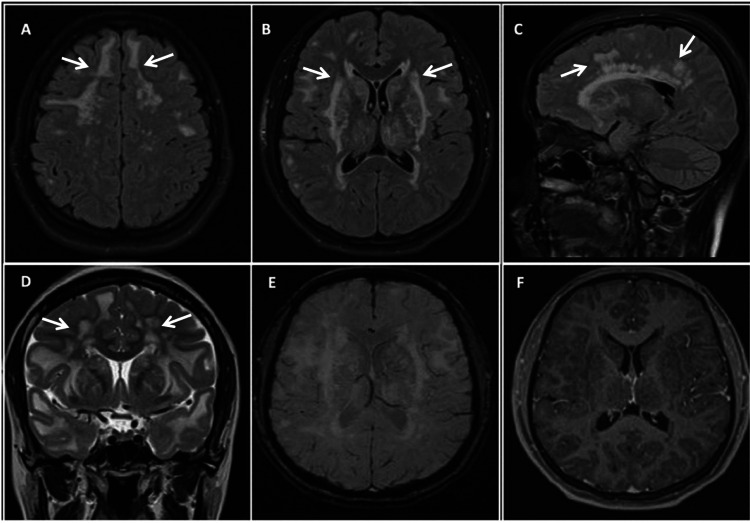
Brain MRI showing axial FLAIR (a and b), sagittal FLAIR (c), and coronal T2 (d) images showing white matter bilateral and symmetrical hyperintensities (white arrows) with a periventricular and subcortical distribution affecting the frontal, anterior temporal, parietal, and external capsule white matter. Axial SWI (e) shows no blooming artifact (microbleed). Post-gadolinium T1 (f) shows no abnormal enhancement MRI: magnetic resonance imaging, FLAIR: fluid-attenuated inversion recovery, SWI: susceptibility weighted imaging
